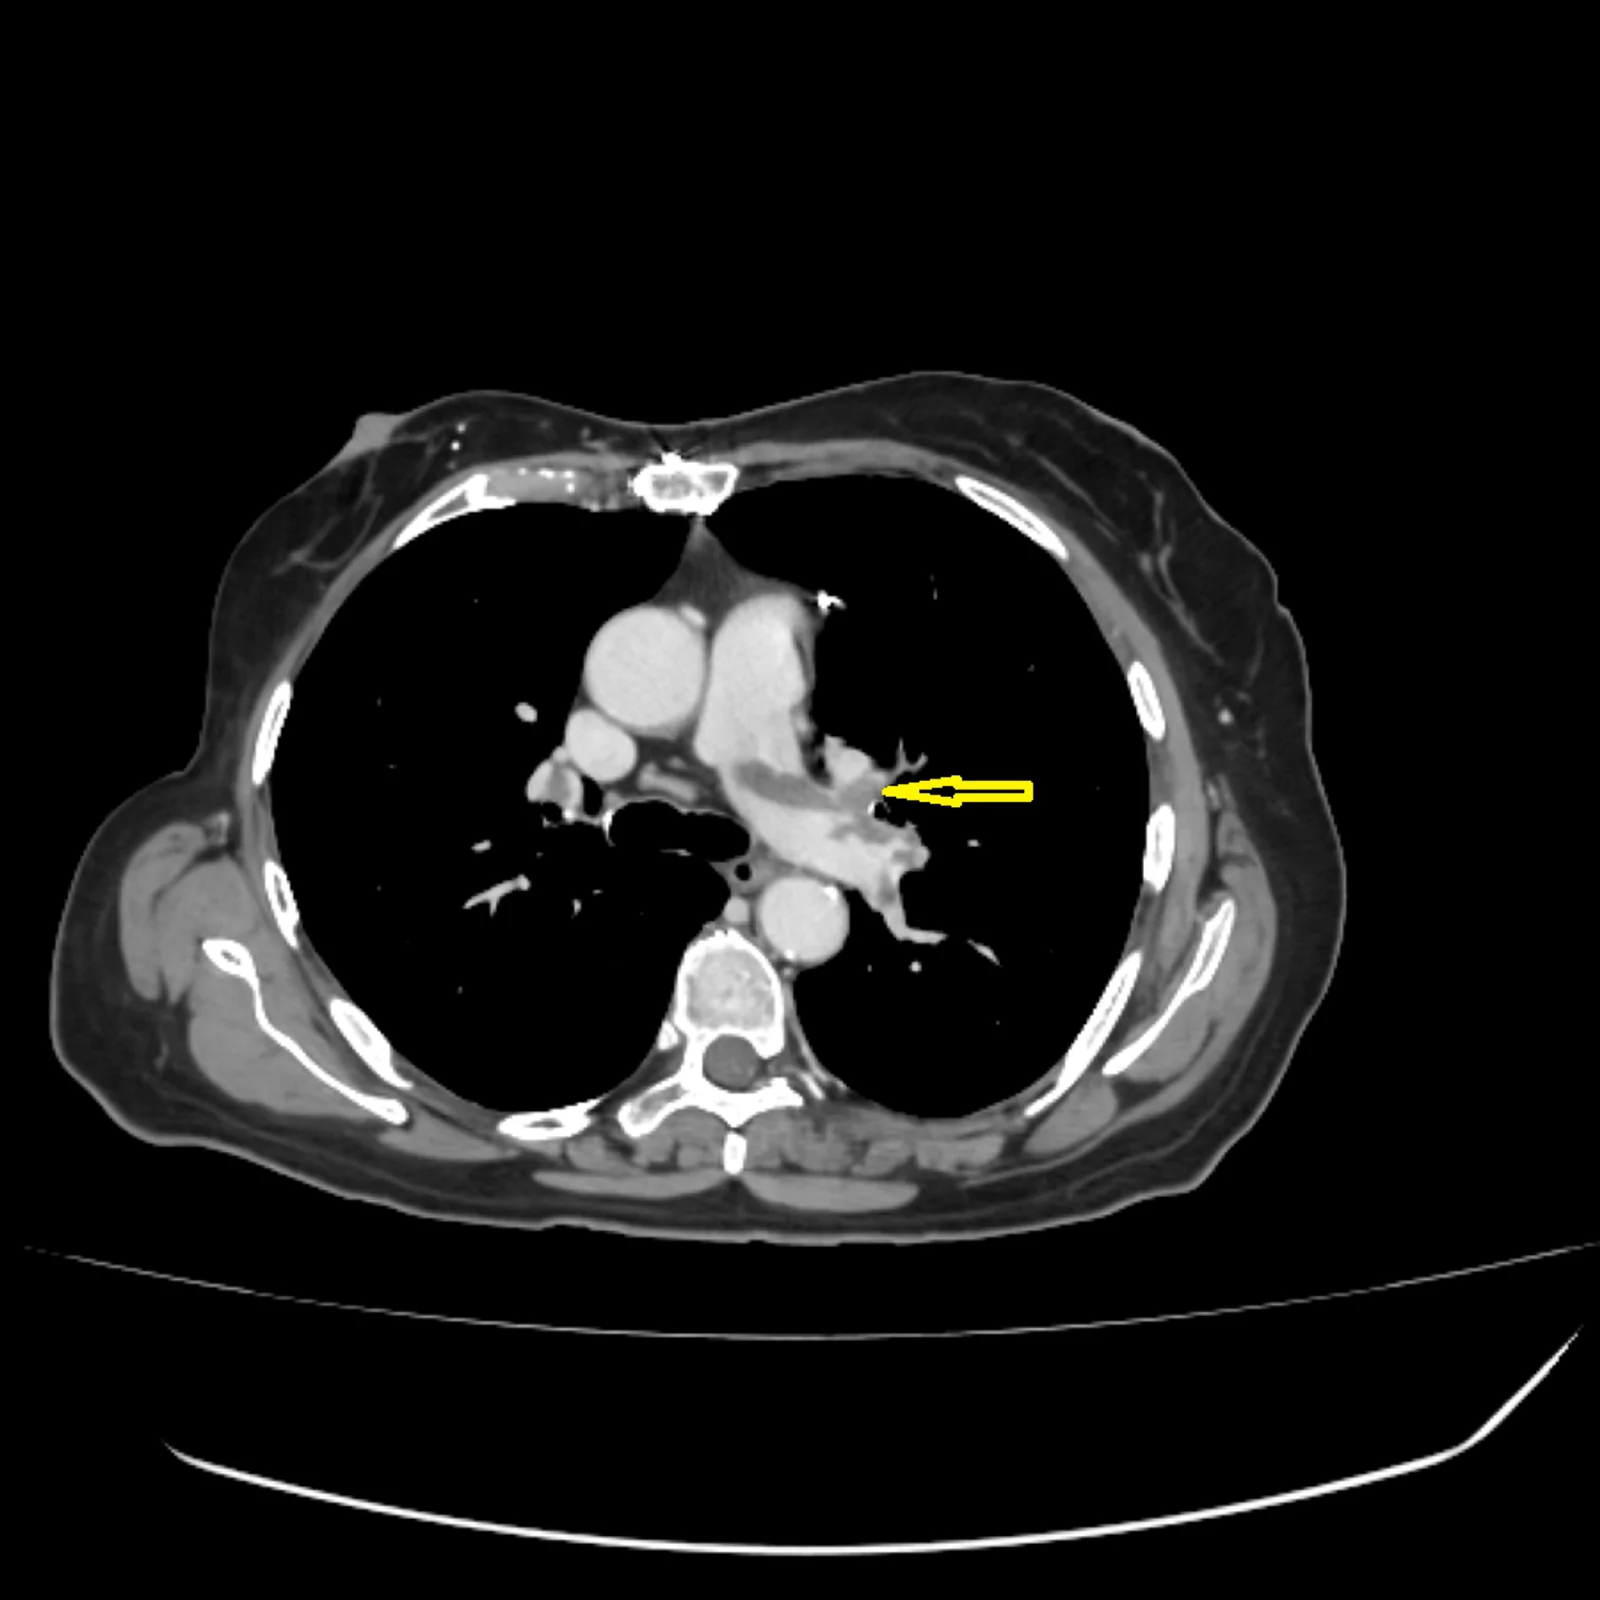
Cross-sectional image of CT thorax with contrast, the yellow arrow pointing towards saddle pulmonary embolism.
